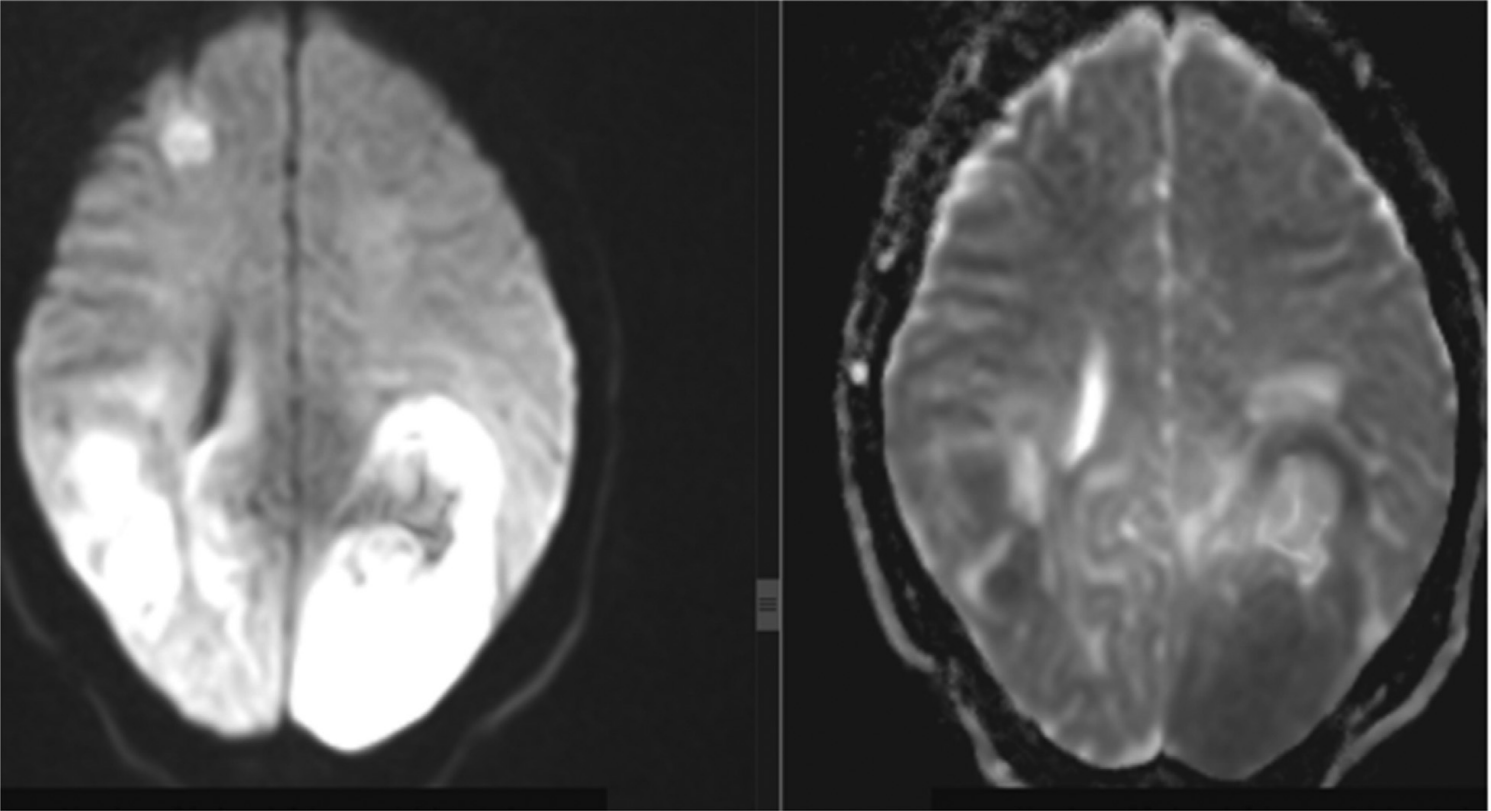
From left to right, the images show DWI and ADC maps. These maps reveal significant diffusion restriction in the lesions.
